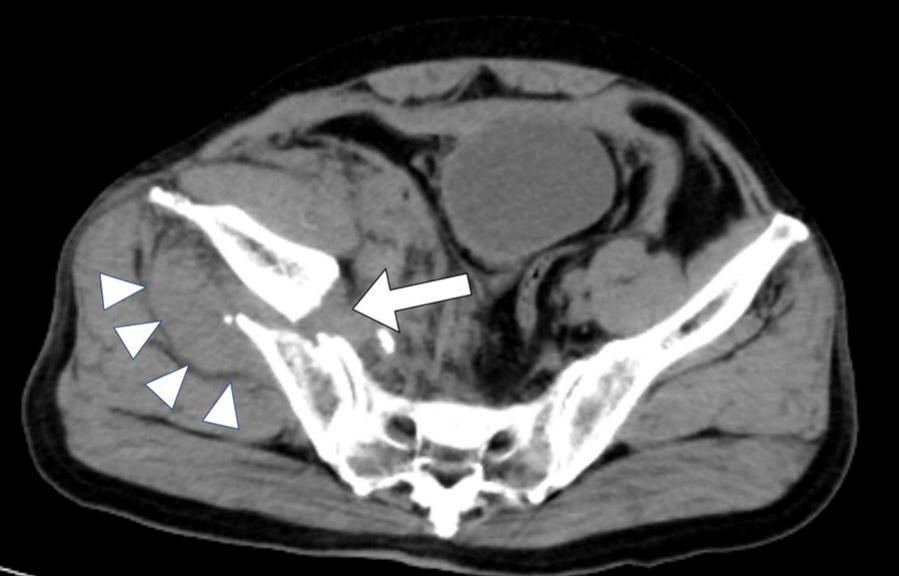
CT findings on day 1. CT revealed a right pelvic fracture (arrow) and a haematoma (arrowhead) in the pelvic extraperitoneal space
